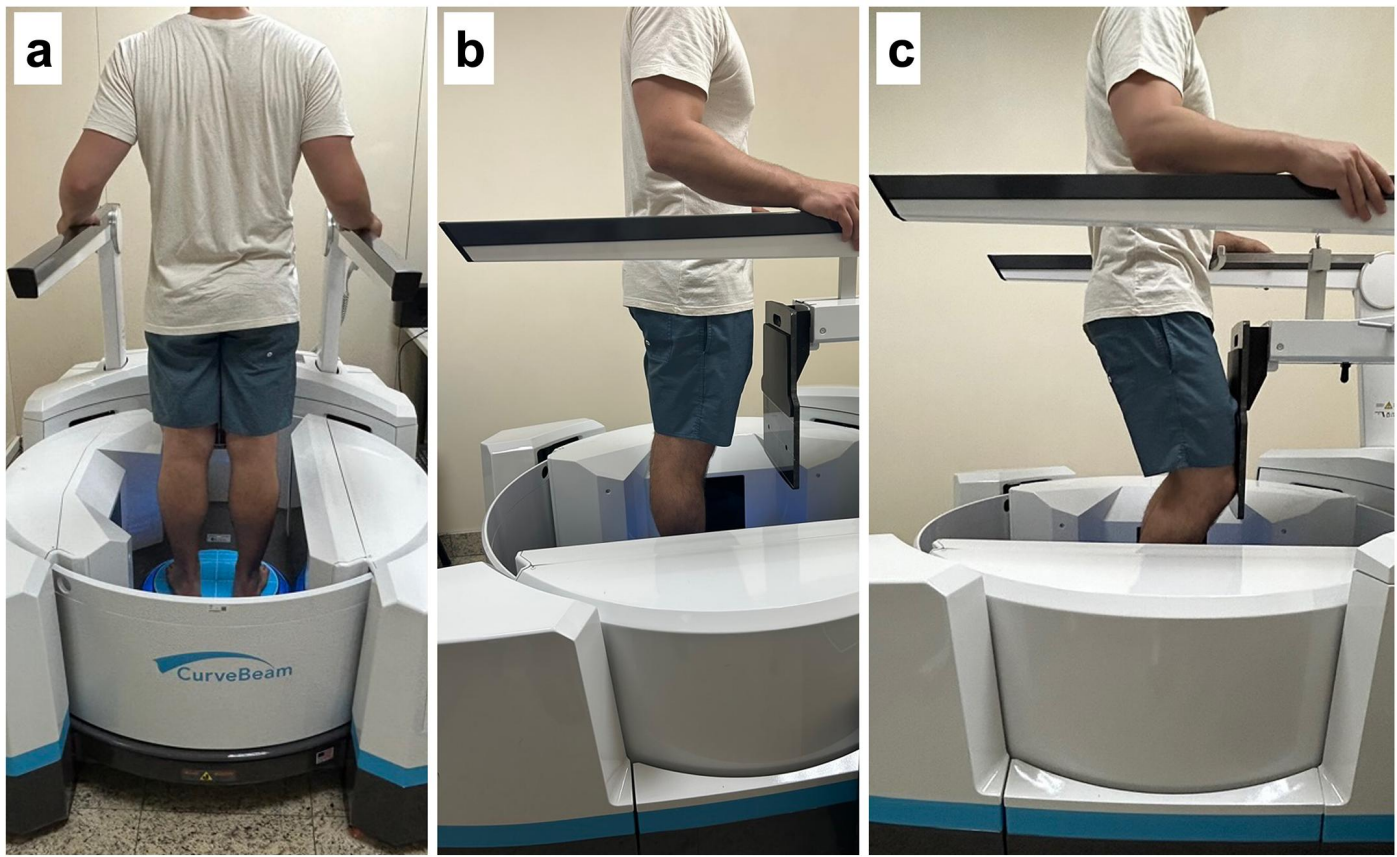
WBCT acquisition protocol for patients with anterior cruciate ligament injury (curveBeam LineUP scanner). (a) Posterior view of the patient standing with the knee in full extension in the cone-beam CT scanner. (b) Lateral view of the patient standing with the knee in full extension in the cone-beam CT scanner. (c) Lateral view of the patient standing with the knee flexed to 30° in the cone-beam CT scanner. Informed consent for publication of the image was obtained from the patient.
